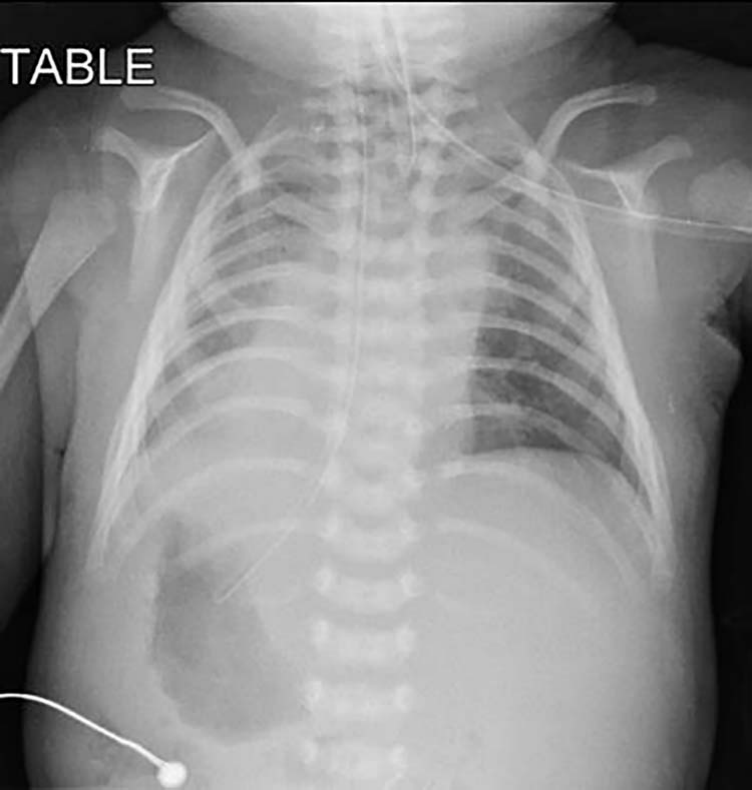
Pre-operative chest radiograph showed apparent dextrocardia and visceral situs inversus (evidenced by lower tip of feeding tube in the right-sided stomach with left-sided homogenous liver opacity).
